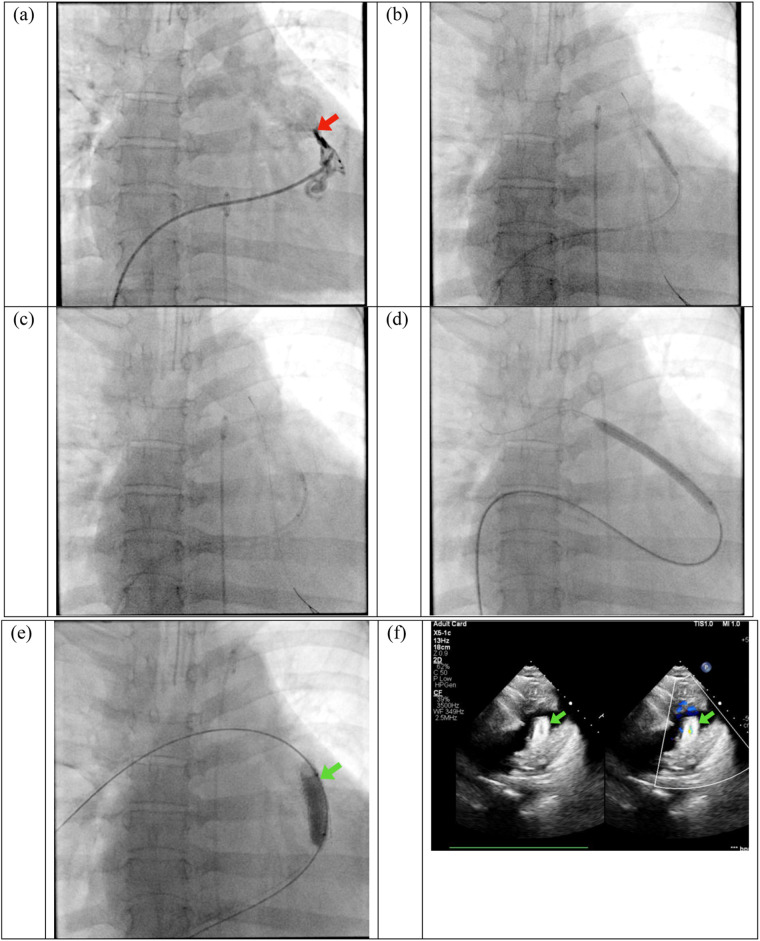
Right ventricular outflow tract (RVOT) stenting in the present patient. (a) Angiogram demonstrating severe RVOT stenosis with nearly absent pulmonary artery flow (*red arrow*). (b) Lesion predilation with a 4.0 × 18 mm Sapphire coronary balloon. (c) Balloon-assisted catheter advancement technique. (d) Inflation with a 6.0 × 60 mm Mustang vascular balloon. (e) Deployment of a 10.0 × 29 mm Omnilink vascular stent. (f) Post-procedural echocardiography confirming appropriate stent position (*green arrow*).
